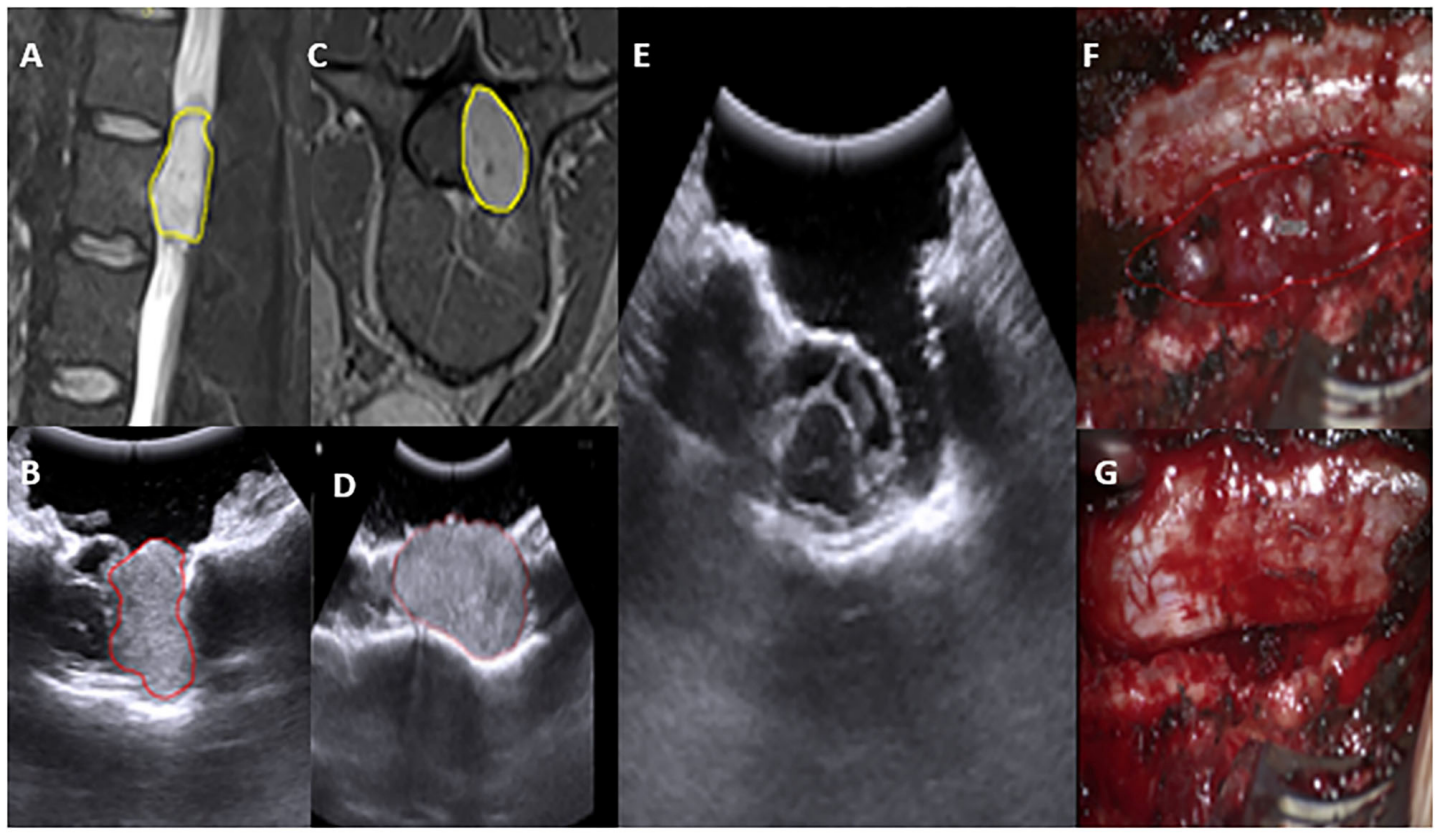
Preoperative MRI T2WI sagittal and axial view depicting an extradural L2–3 lesion (A,C) yellow. In concordance, IUS depicting the lesion-location with spinal cord boundary (B,D). Post-operative IUS Clearly shows total resection (E). Intraoperative images, prior and post-resection (F,G).
